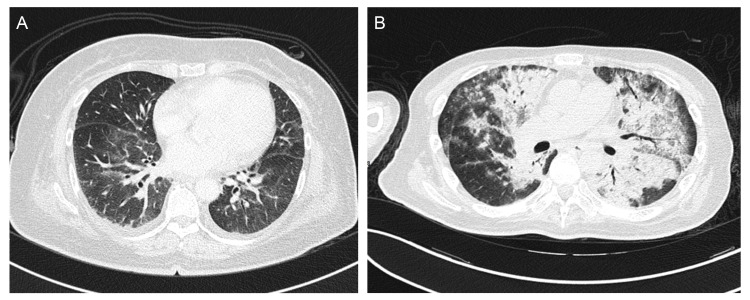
Patient 1’s high-resolution computed tomography (HRCT) images (Figure 2A) show mild reticulations involving bilateral lower lobes, suggestive of interstitial lung disease. Patient 2’s HRCT images (Figure 2B) reveal a diffuse air-space filling process characterized by consolidation mixed with some ground-glass opacities involving both the central lungs, sparing the peripheral parenchyma, and consistent with the butterfly pattern; this is indicative of acute pulmonary alveolar edema.
